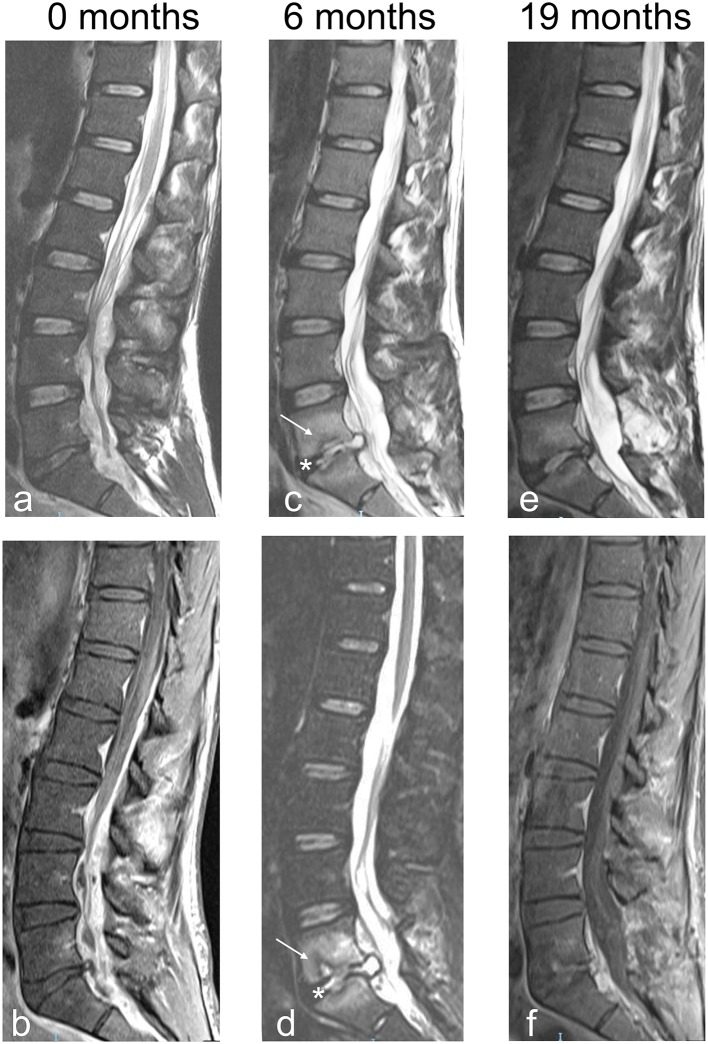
Sagittal MRI images of patient no. 2. (a) Sagittal MRI T2 weighted image obtained the day of surgery. There is a lack of obvious involvement of the bone despite a large SEA that circumferentially compresses the dural sac from L2 to S1. (b) Sagittal MRI T1 weighted with contrast. Same as in (a). (c) Sagittal MRI T2 weighted image obtained 6 months after L5 laminectomy and SEA evacuation. Alterations compatible with PS involving the vertebral bodies of L4 and L5 (arrow) and the intervening disk (asterisk) are now visible. (d) Sagittal MRI T1 weighted with contrast. Same as in (c). (e) Sagittal MRI T2 weighted image with contrast obtained 19 months after L5 laminectomy and SEA evacuation. Alterations related to PS have much regressed, without significant vertebral deformity. (f) Sagittal MRI T1 weighted with contrast. Same as in (e).
